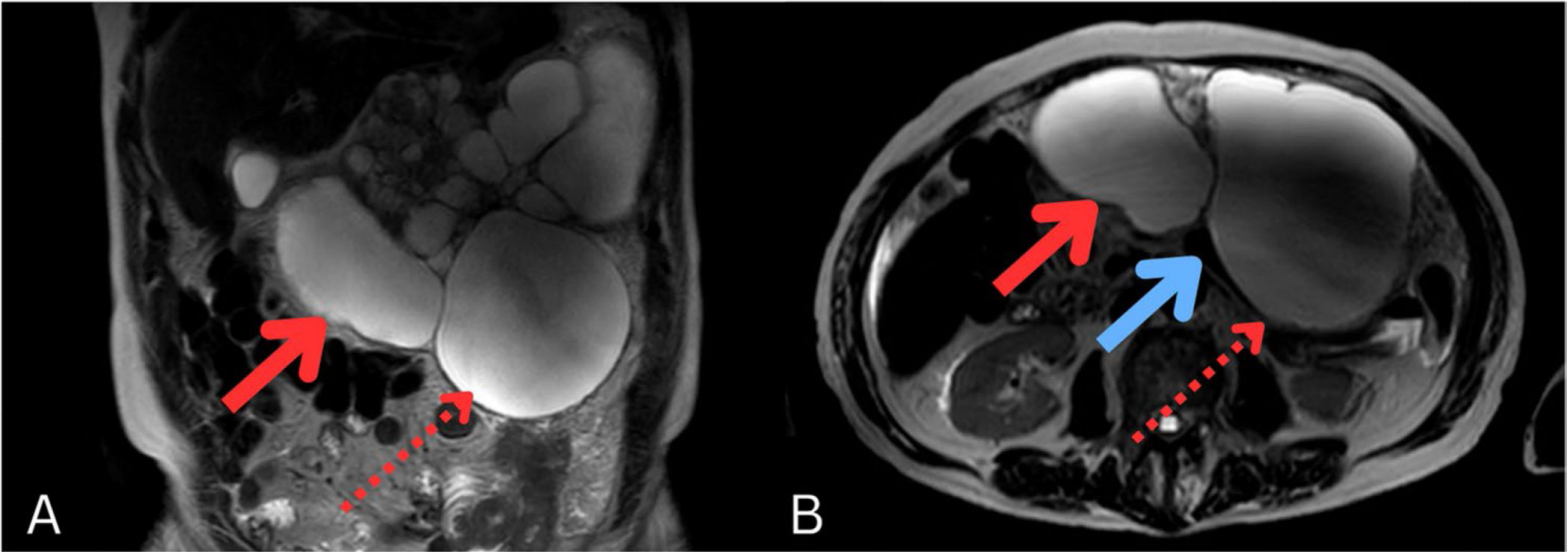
Preoperative MRI revealed A, B a large multiloculated cystic epigastric mass, measuring 21.2 cm × 12.6 cm × 18.6 cm, with two dominant cysts: red solid arrow points to smaller proximal cyst and red dotted arrow points to larger distal cyst. B The mass was contiguous with the pancreatic neck, causing pancreatic duct narrowing (blue solid arrow)
